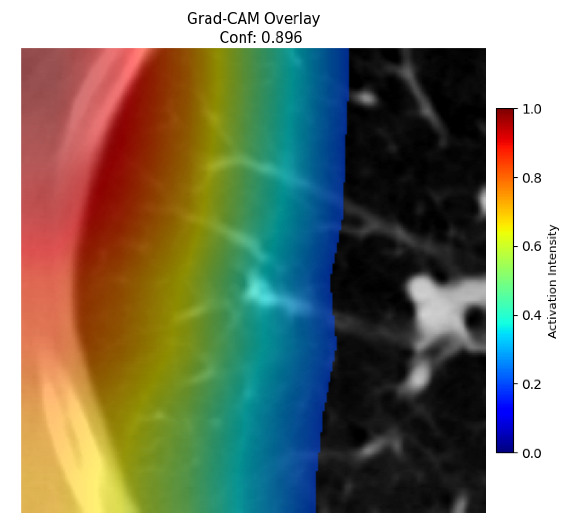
Table 6 bioengineering-13-00552-t006: LIME and Grad-CAM explanations generated by four lightweight CNN variants using EfficientNetB0 as backbone, applied to four representative CT images.
